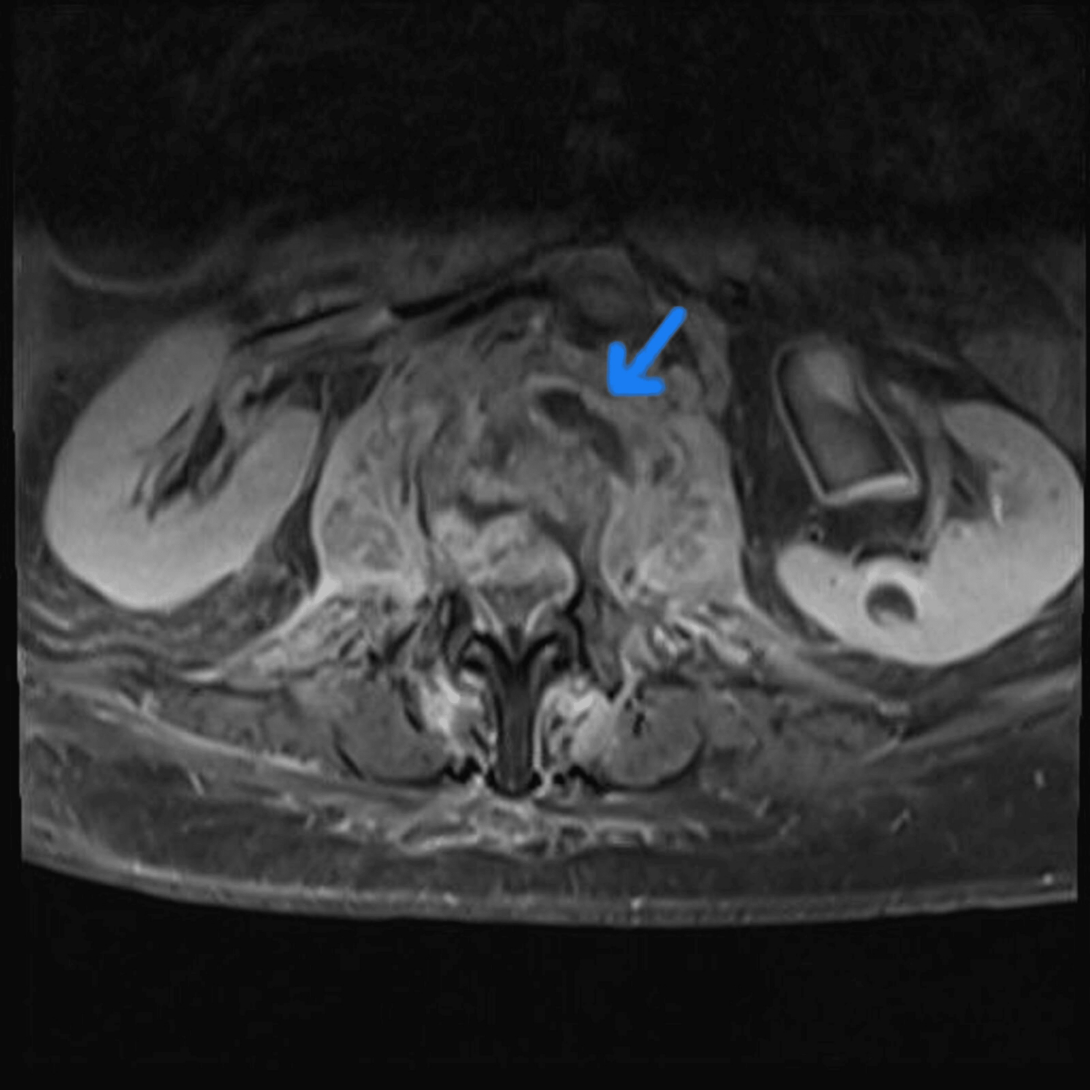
MRI lumber spine showing complete replacement of the L1 and L2 vertebral bodies by high signal, and a newly identified epidural abscess narrowing the spinal canal and indenting the conus medullaris.
